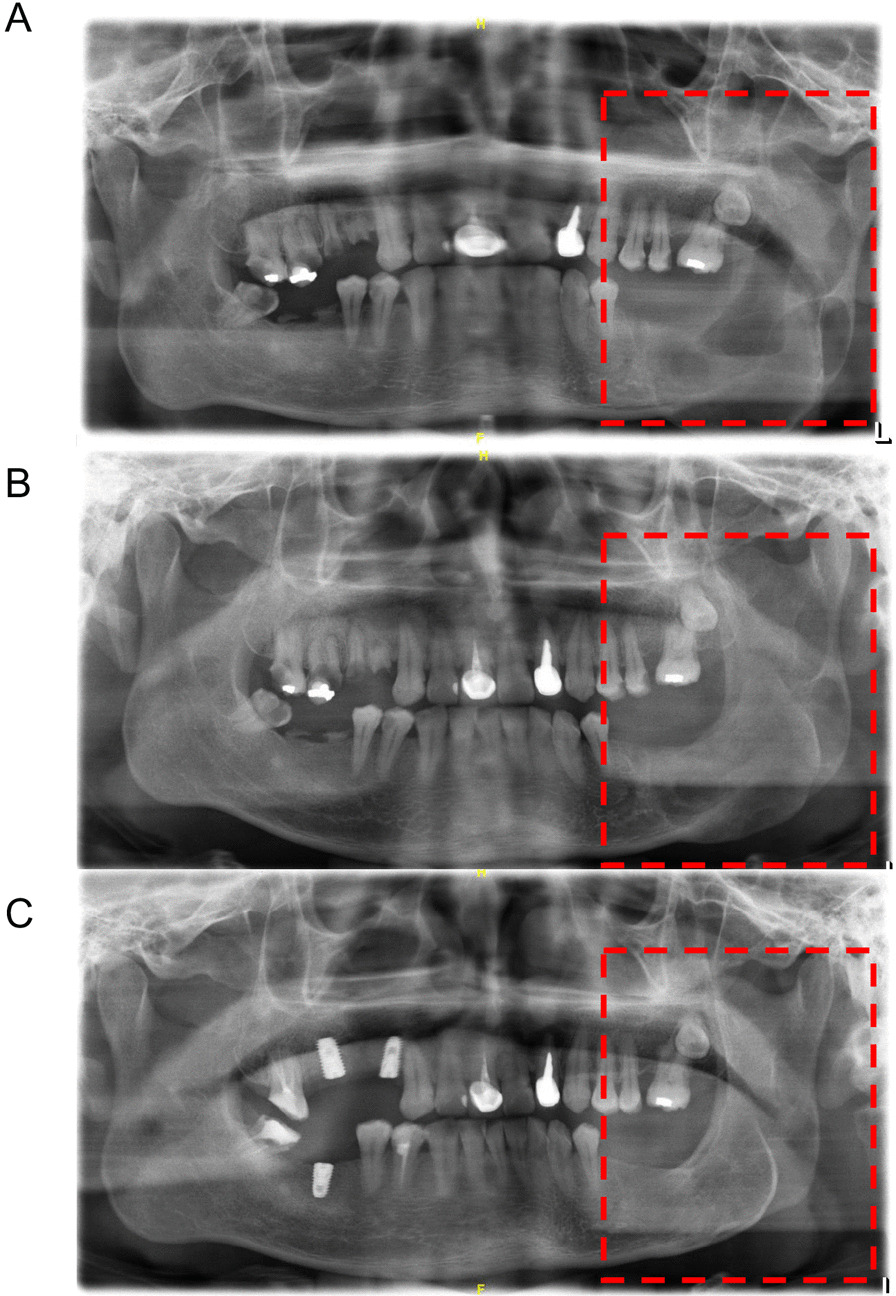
Comparison of pre-operative and post-operative results of FDSC strategy for MA patients. a Pre-operative panoramic radiography of patients; b The cystic cavity was reduced half a year after fenestration decompression; c The cystic cavity disappeared 2 years after secondary curettage
